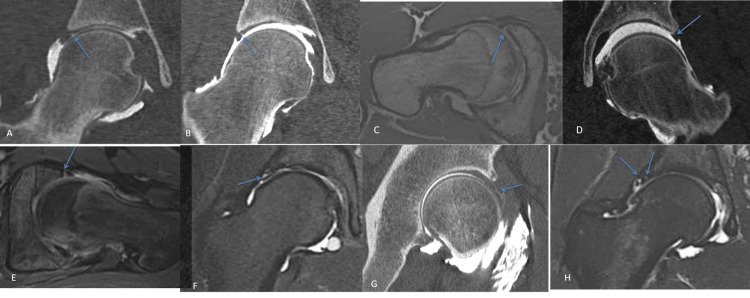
Different kinds of labral lesions (A) Labral-chondral separation in computed tomography arthrography (CTA) without traction and (B) with traction. (C) Partial labral tear in CTA with traction and (D) magnetic resonance arthrography (MRA). (E) Complete labral tear in MRA. (F) Intrasubstance labral tear in MRA and (G) CTA without traction. (H) Complex labral tear in MRA
